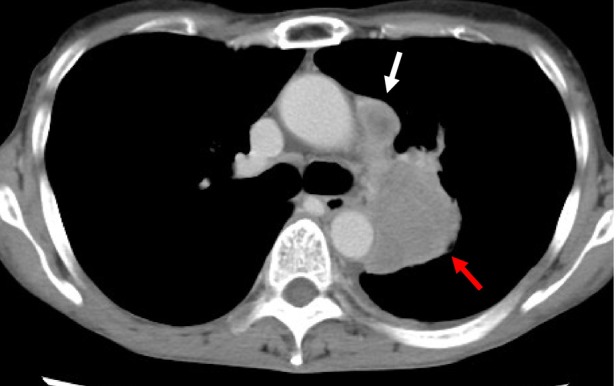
Computed tomography (CT) showing a mass in the left lung (red arrow), lymph node swelling (white arrow), and small amount of pleural effusion (A).
